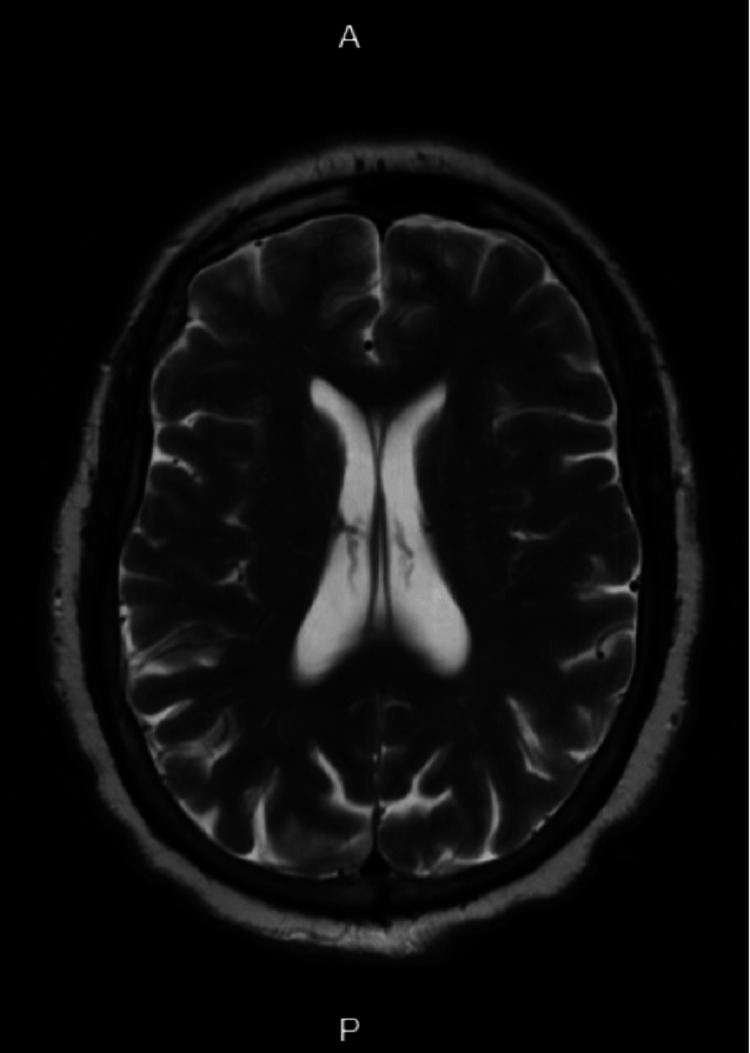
MRI brain A: Anterior; P: Posterior
